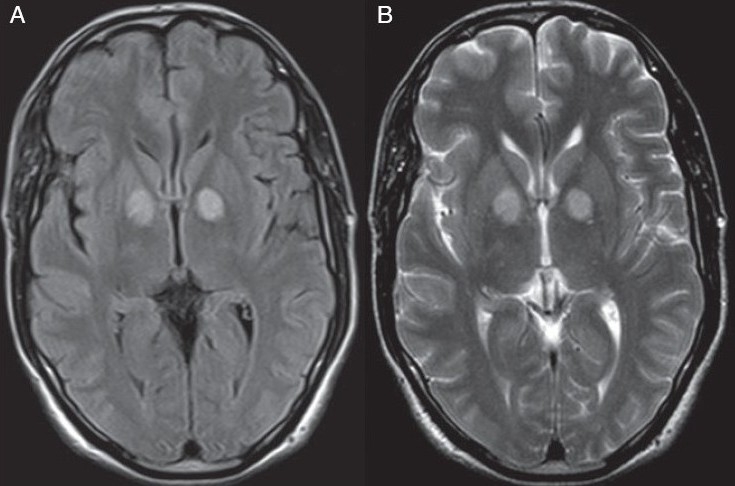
(A) FLAIR axial image showing bilateral symmetrical basal ganglia lesions (B) T2 weighted axial MR image showing bilateral symmetrical basal ganglia lesions
